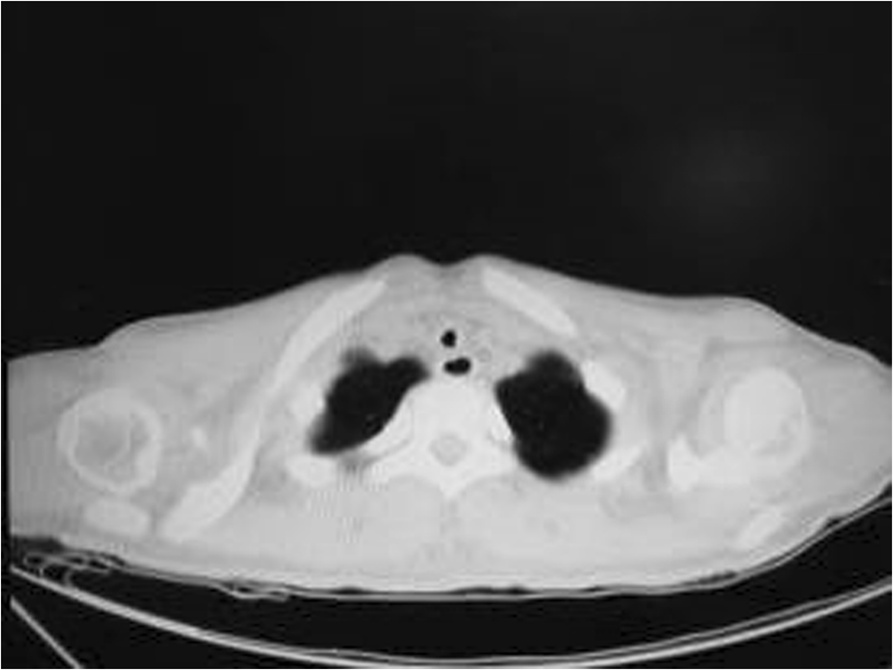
A chest computed tomography scan showing tracheal stenosis at thoracic inlet before treatment in Case 1
